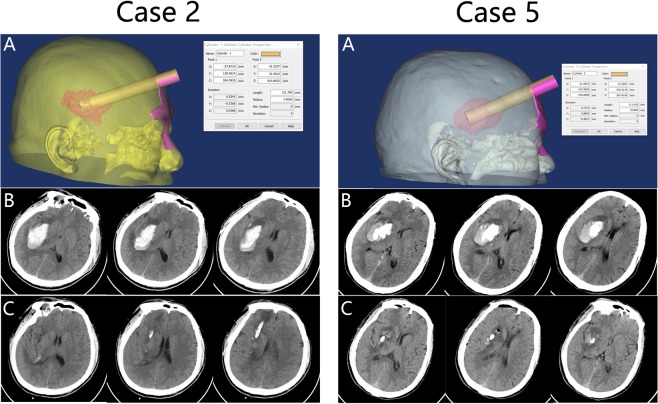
(A) 3D reconstruction and computer-assisted planning were used to determine the trajectory of the puncturing needle. Preoperative (B) and postoperative (C) CT scans for case 2 and 5 in this study.
